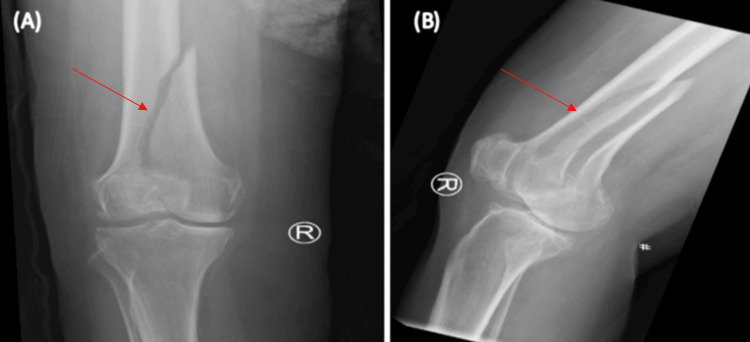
Initial X-ray upon presentation (A) An anterior-posterior (AP) view and (B) a lateral view. The initial X-ray upon presentation showed a displaced medial femoral condyle, with a fracture line from intercondylar notch extending to the diaphysis, and a non-displaced lateral femoral condyle.
